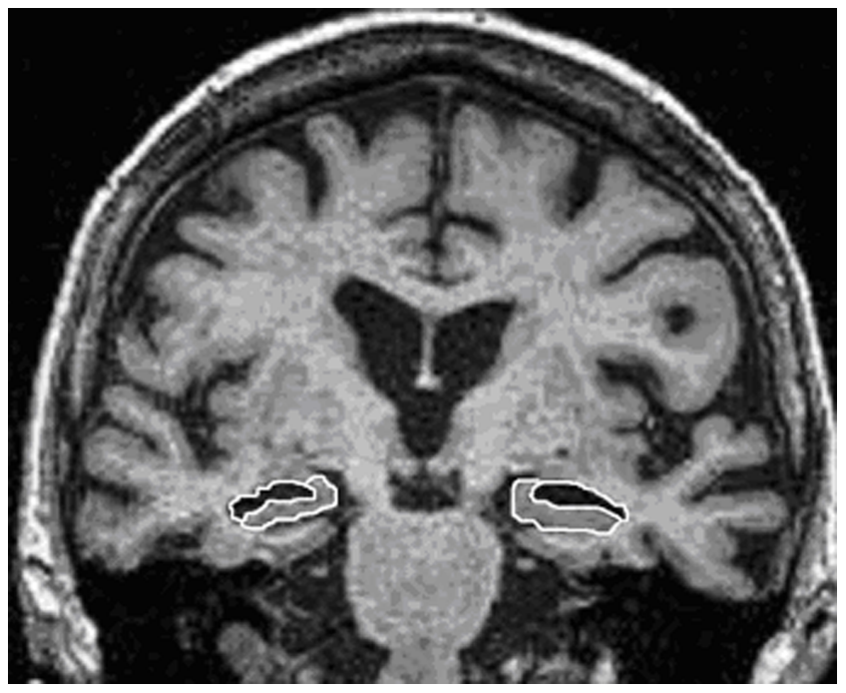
Example of manual anatomical delineation of the hippocampus (white line with grey area inside) and temporal horn of the lateral ventricle (white line with black area inside) on the left and right side. Coronal sections of the MRI are located at the transition from the *alveus* of the hippocampus into the hippocampal body.
